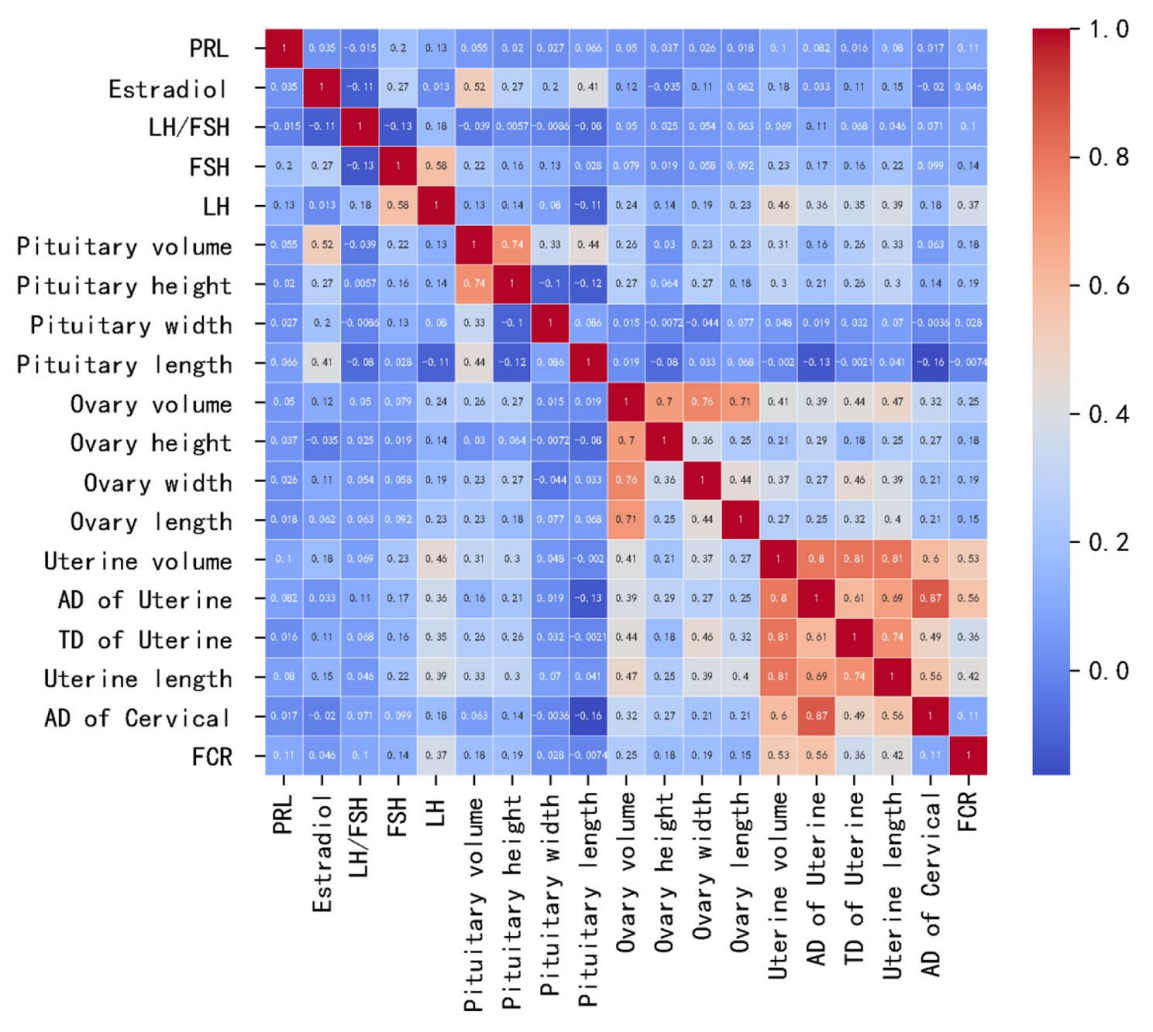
Correlation of the pelvic ultrasound and pituitary MRI parameters with pubertal hormone levels in ICPP group. ∗Values in black font represent statistically significant correlations. Ovarian parameters are mean values for bilateral ovaries Abbreviations: PRL: prolactin; LH, luteinizing hormone; FSH, follicle-stimulating hormone; TD, Transverse diameter; AD, Anteroposterior diameter; FCR, Ratio of fundus to cervix
